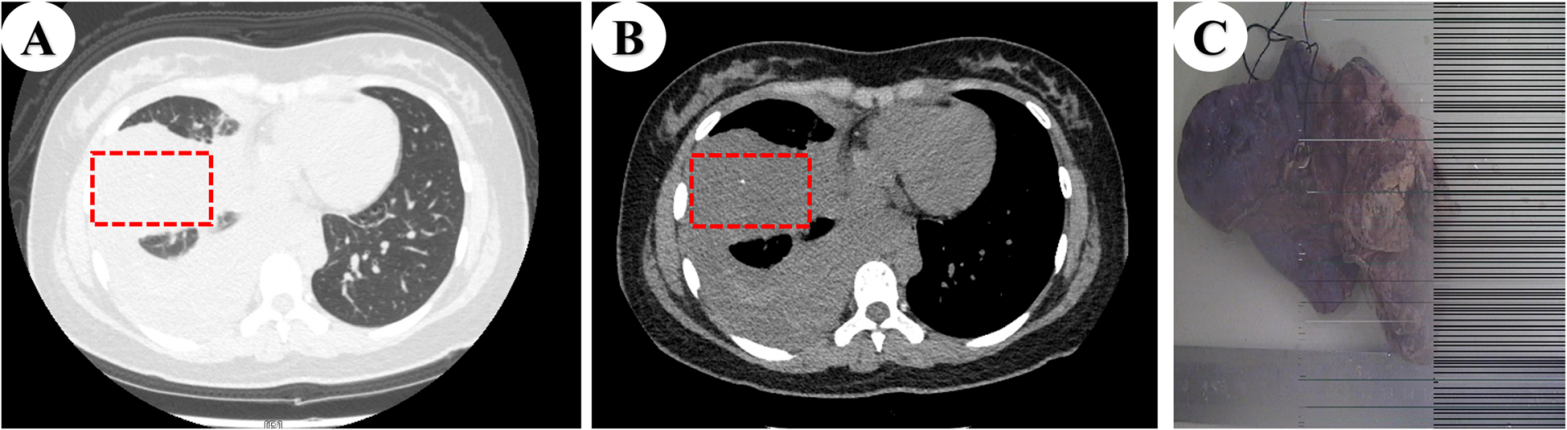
a, b Computed tomography chest axial images showing massive right pleural effusion, right lung collapse, and punctate calcification. c The macroscopic pathological picture of lump in the upper right lobe
